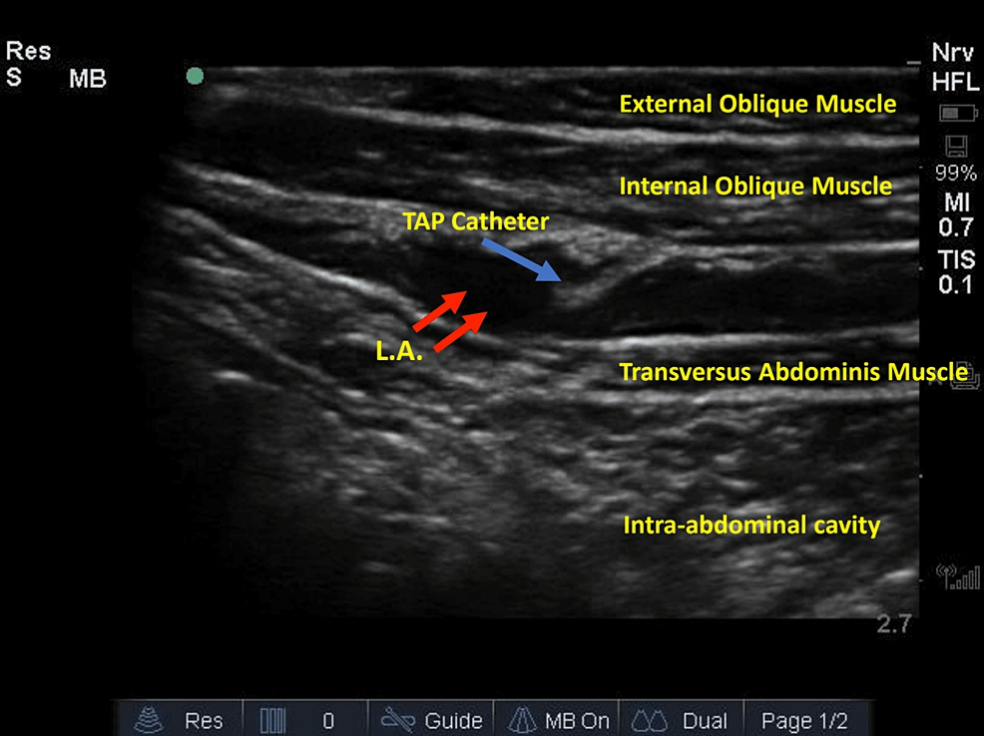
Ultrasonic anatomy and for the placement of TAP catheters done directly on abdominal musculature. No skin or subcutaneous tissue can be appreciated. The blue arrow shows the TAP catheter, and the red arrows show the spread of local anesthetic in the plane between transversus abdominis and internal oblique muscles. TAP -  transversus abdominis plane, LA - local anesthetic
